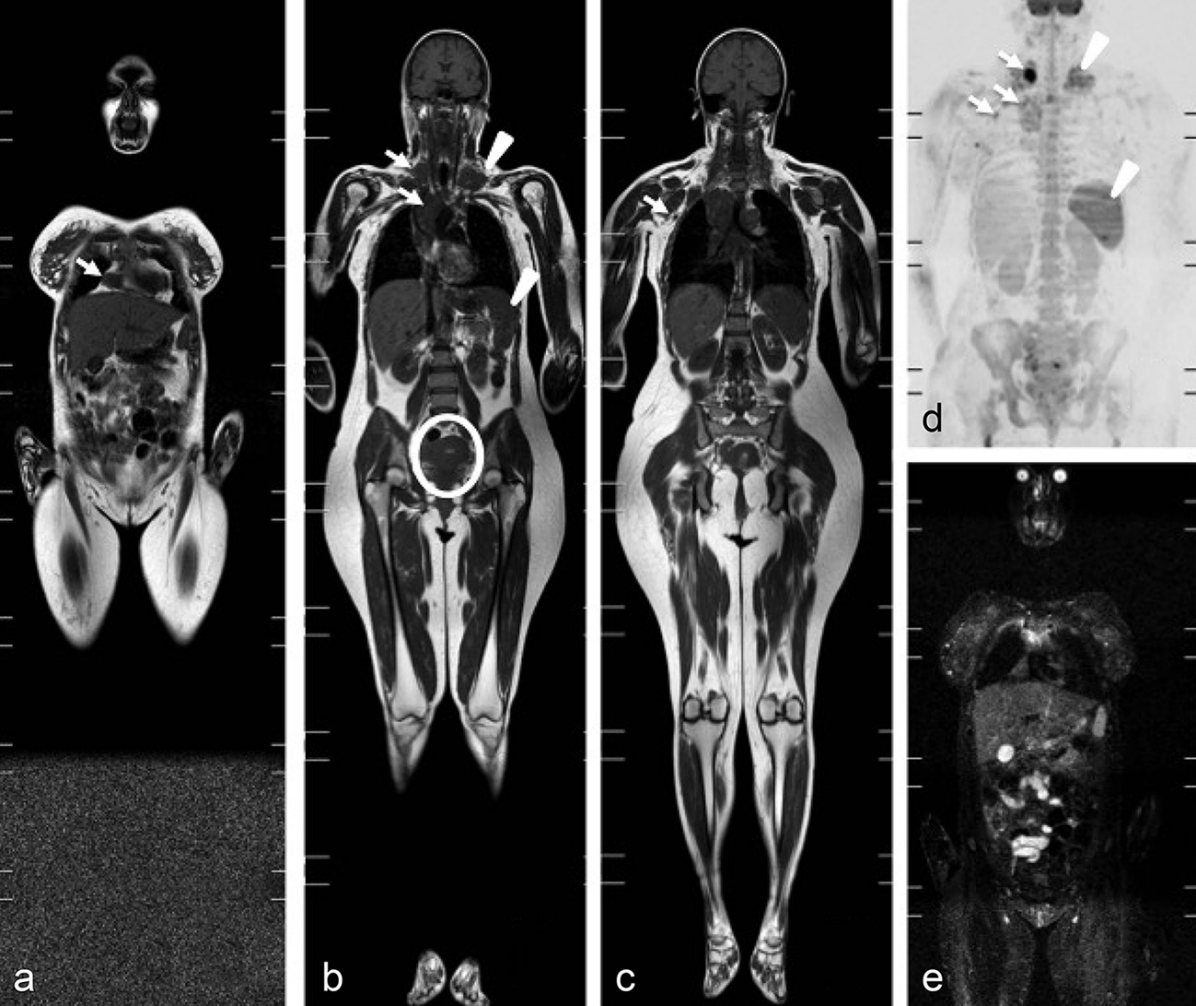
Images from scanning performed 2 weeks after delivery. Coronal *T*_1_ weighted (a–c), coronal maximum intensity projection diffusion-weighted (d) and coronal short tau inversion-recovery (e) whole-body MRI showing dimensional decrease of the axillary, cardiophrenic, right supraclavicular and mediastinal lymph nodes (a–d; arrows), a slight enlargement of the spleen and of left supraclavicular lymphadenopathy (b, d; arrowheads). Note also a still enlarged uterus after delivery (b; circle).
